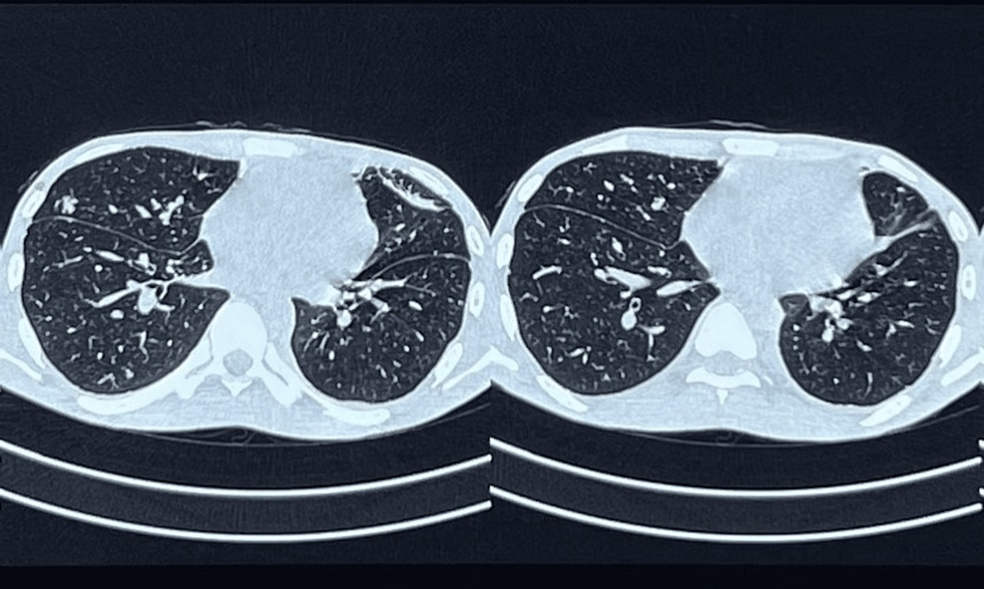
CT of the chest showing multiple ill-defined nodules
